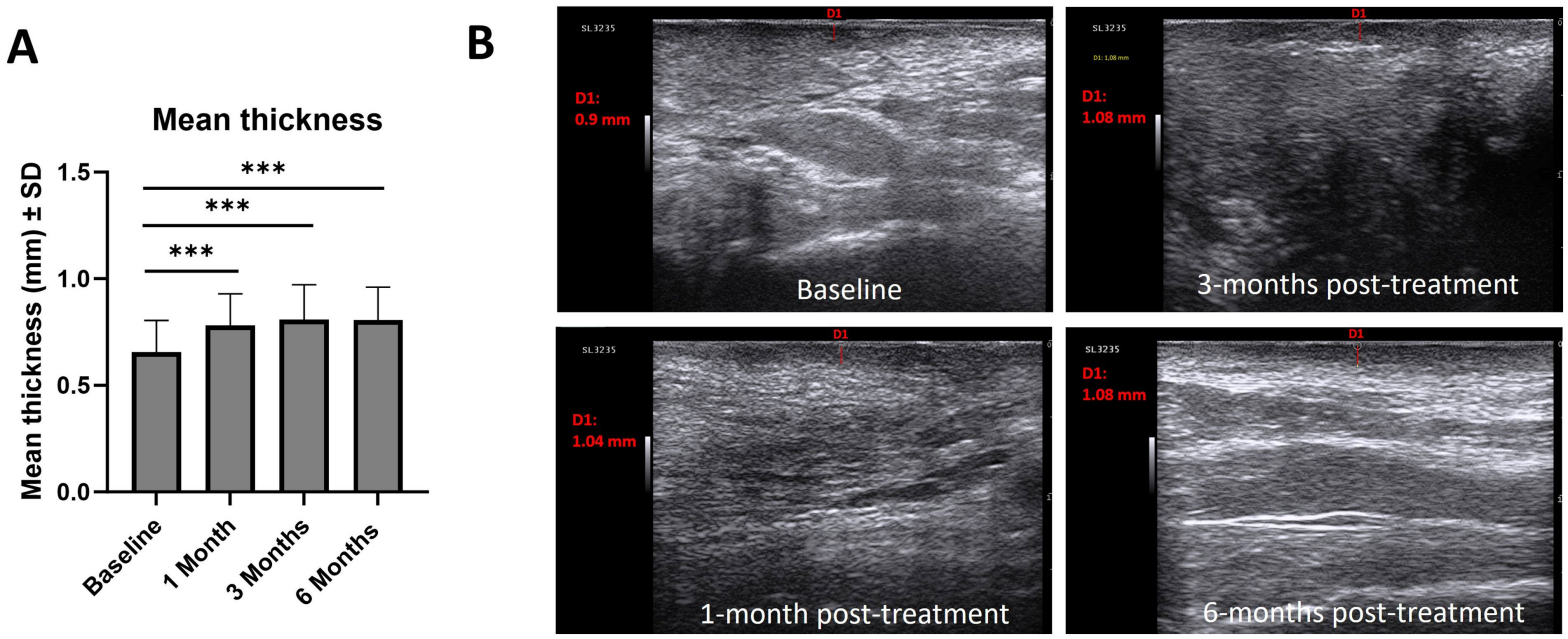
(A) Mean thickness (mm, ± SD) of the hypoechoic band of the dermal-epidermal junction and ultrasound images of the hypoechoic band, (B). Example of 3 ultrasound measurements of hypoechoic band thickness. Analysis conducted using Wilcoxon matched-pairs signed rank test: p* < 0.05, *p* < 0.01, p* < 0.001. SD, standard deviation.
